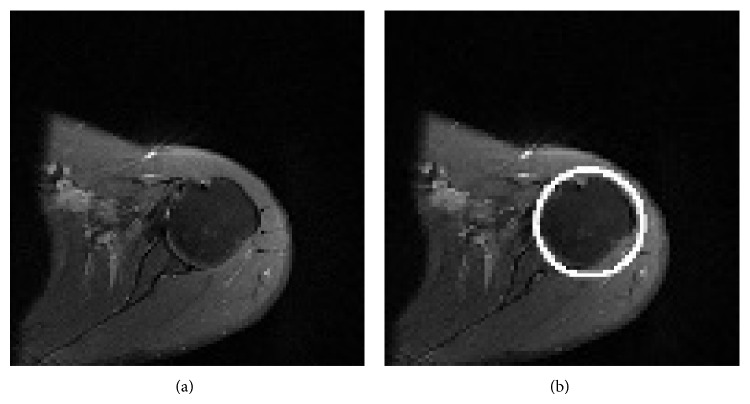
(a) Orginal MR image of left shoulder and (b) detection of humeral head by using the circular Hough transform.
